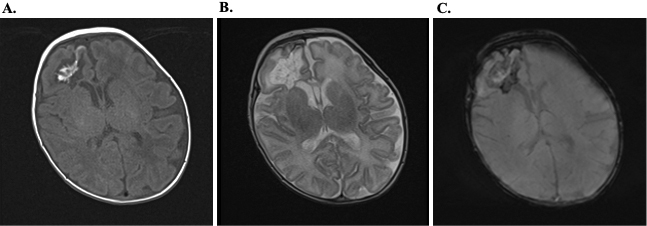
Head MRI. Head magnetic resonance imaging on day 46 showed DMVT and associated peripheral post-infarction changes, such as cystic encephalomalacia, confirming cerebral venous infarction. Axial T1-weighted images demonstrate linear hyperintense lesions in right frontal lobe (A). The corresponding T2-weighted image shows linear hypointense alterations at the same location (B). Susceptibility-weighted imaging did not show any hypointense signals in the affected area, suggesting a thrombotic rather than hemorrhagic process (C). These linear signal changes are suggestive of DMVT. Cystic encephalomalacia is observed in the surrounding parenchyma, indicating secondary tissue degeneration. No thrombosis was observed in the cerebral venous sinuses. DMVT: deep medullary venous thrombosis; MRI: magnetic resonance imaging.
